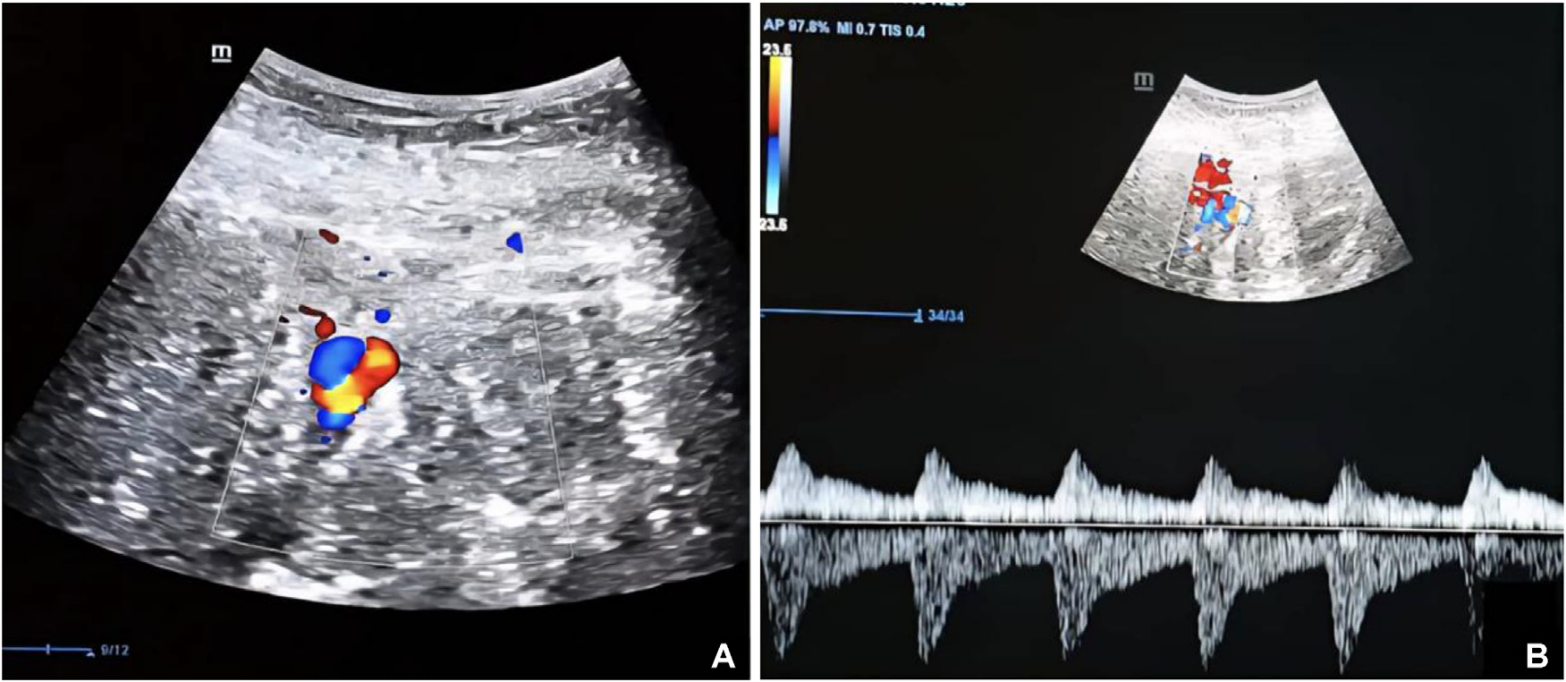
(A) Doppler Ultrasound with characteristic ``Yin Yang sign'' and (B) Pulsed wave Doppler: ``to-and-fro'' pattern refers to a characteristic bidirectional blood flow.
